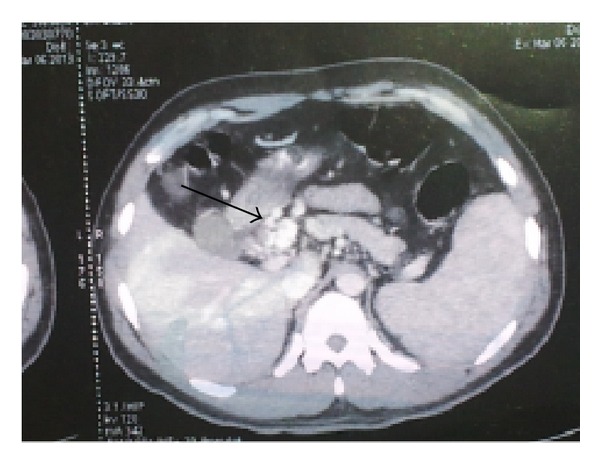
CT angiogram showing a coronal view of multiple collaterals (arrow) with poorly localized portal vein.
